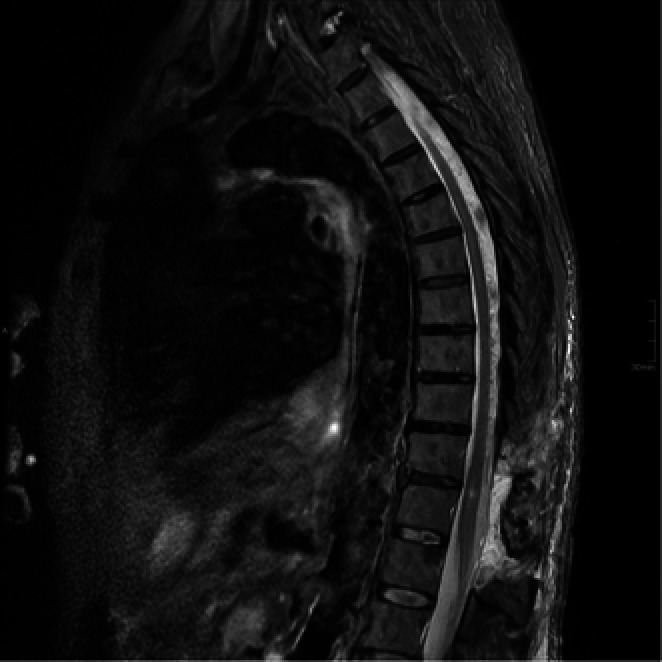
FIGURE 2 Sagittal STIR spinal MRI showing postoperative changes at the level of the previous hematoma, with persistent but reduced extent of myelopathy. Engorged perimedullary vessels surrounding the spinal cord are observed, raising suspicion of an underlying vas.
